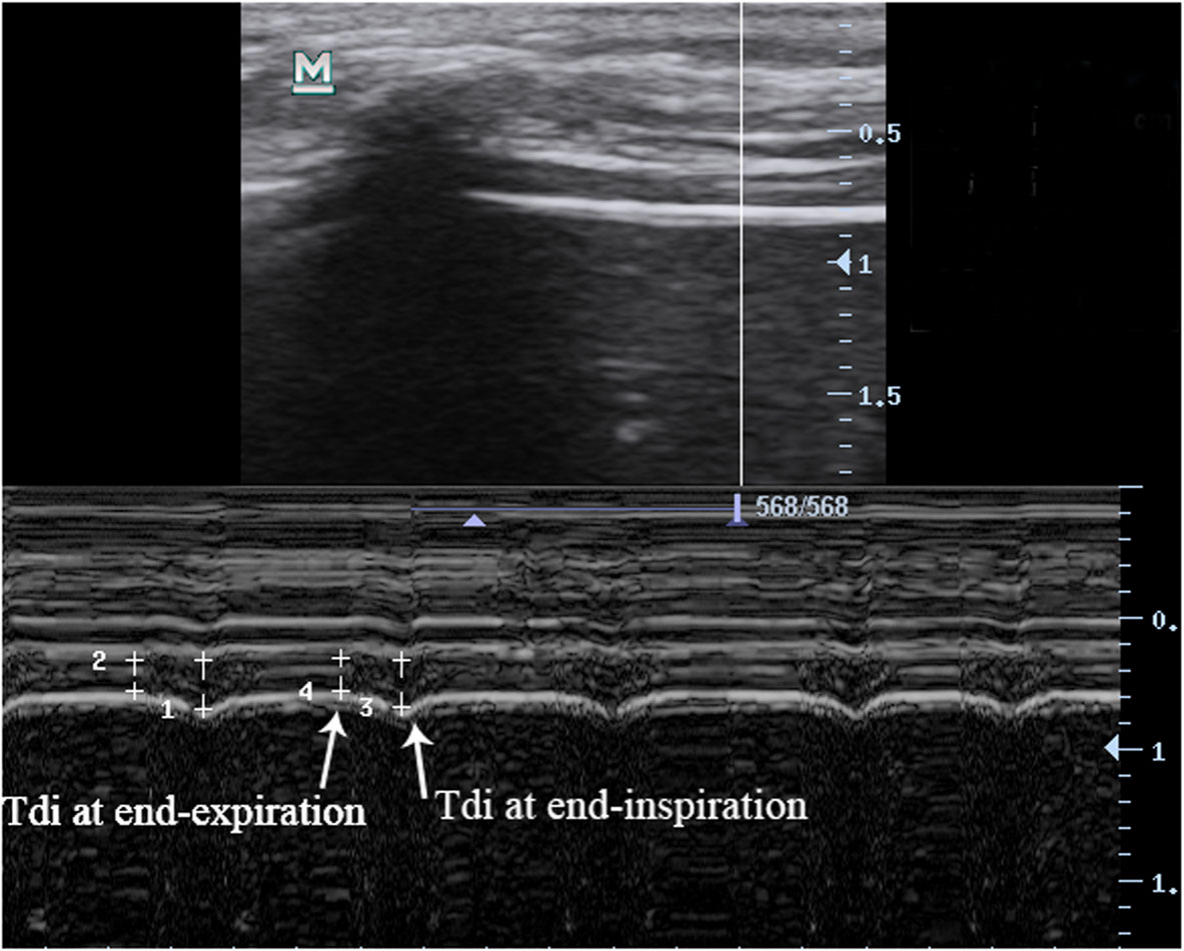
Ultrasound M-mode using a 10 MHz probe in the zone of apposition
